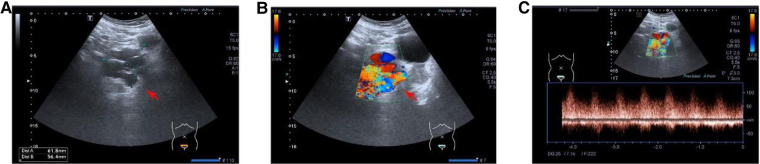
(A) Multiple tortuous tubular structures within an irregularly shaped anechoic lesion were revealed by B-mode ultrasound. (B) Color Doppler flow imaging displayed rich colorful blood flow signals as a colored mosaic pattern. (C) Spectral Doppler showed continuous turbulent flow in the systolic and diastolic phases with a high-velocity low-resistance waveform.
